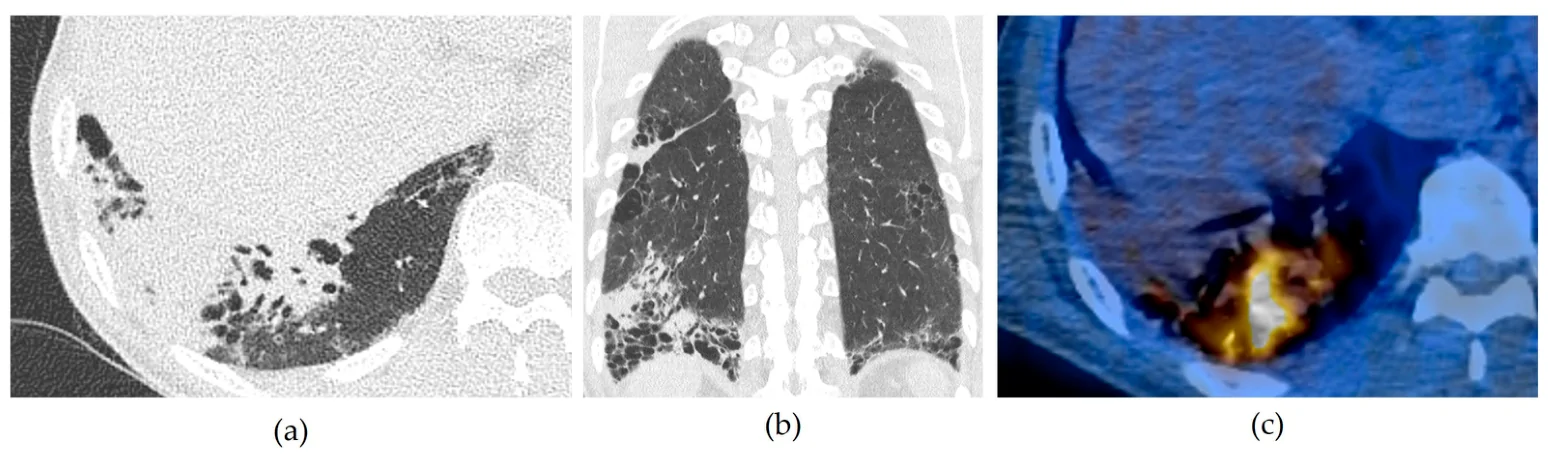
Chest CT images of a 70-year-old man with a 50-pack-year smoking history. (a,b) CT images show a consolidative mass in the right lower lobe, with coexisting usual interstitial pneumonia pattern interstitial lung disease characterized by honeycombing and traction bronchiectasis in both lower lobes. A non-tertiary hospital radiologist categorized the lesion as S (suggestive of pneumonia), whereas a thoracic radiologist at a tertiary hospital assigned a Lung-RADS category 4X. (c) The fluorodeoxyglucose (FDG) PET/CT image shows intense FDG uptake in the RLL mass. The lesion was ultimately confirmed as squamous cell carcinoma on lung biopsy.
